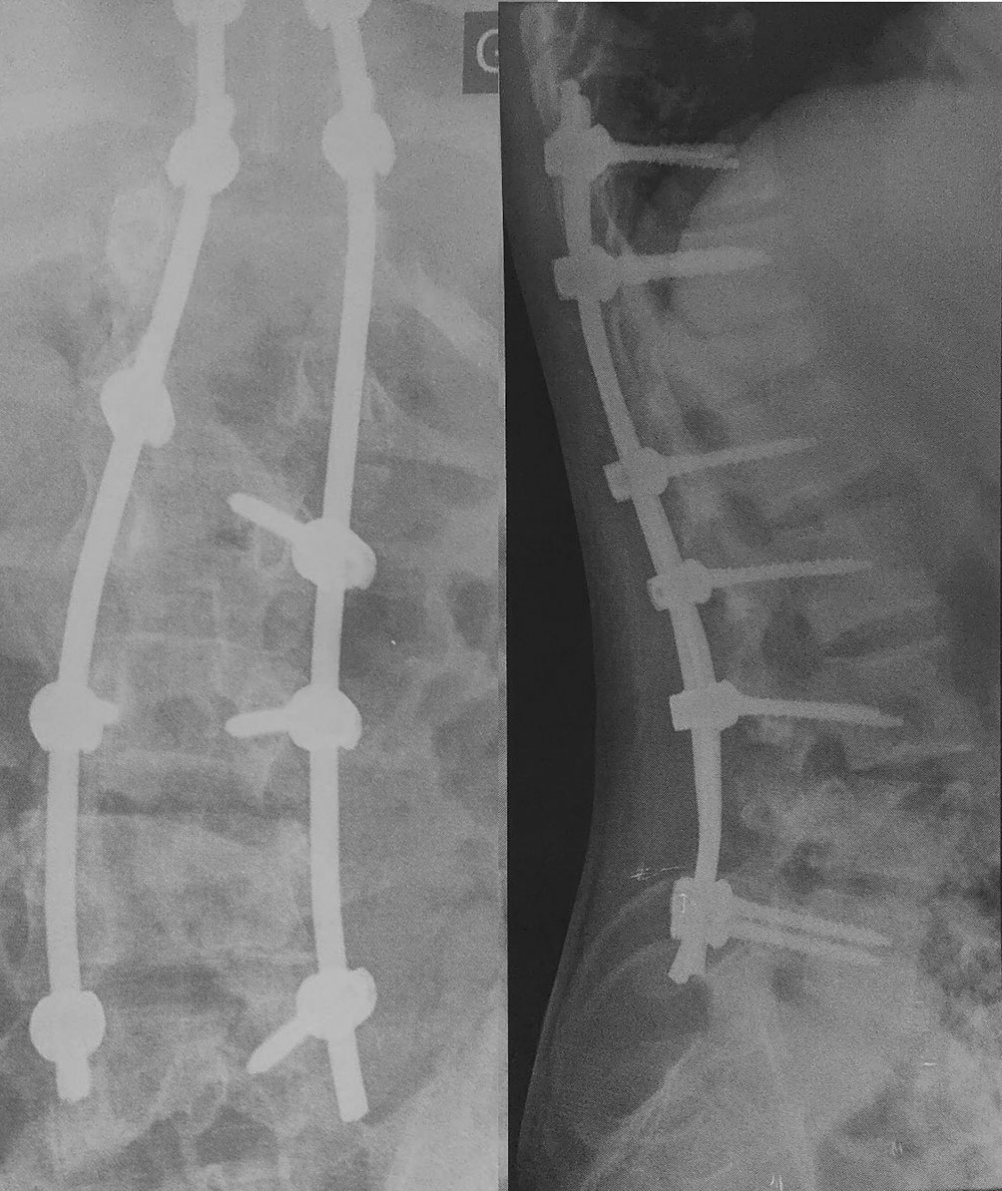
Postoperative anteroposterior and profile radiography of the T10-L5 postero-lateral fusion.
